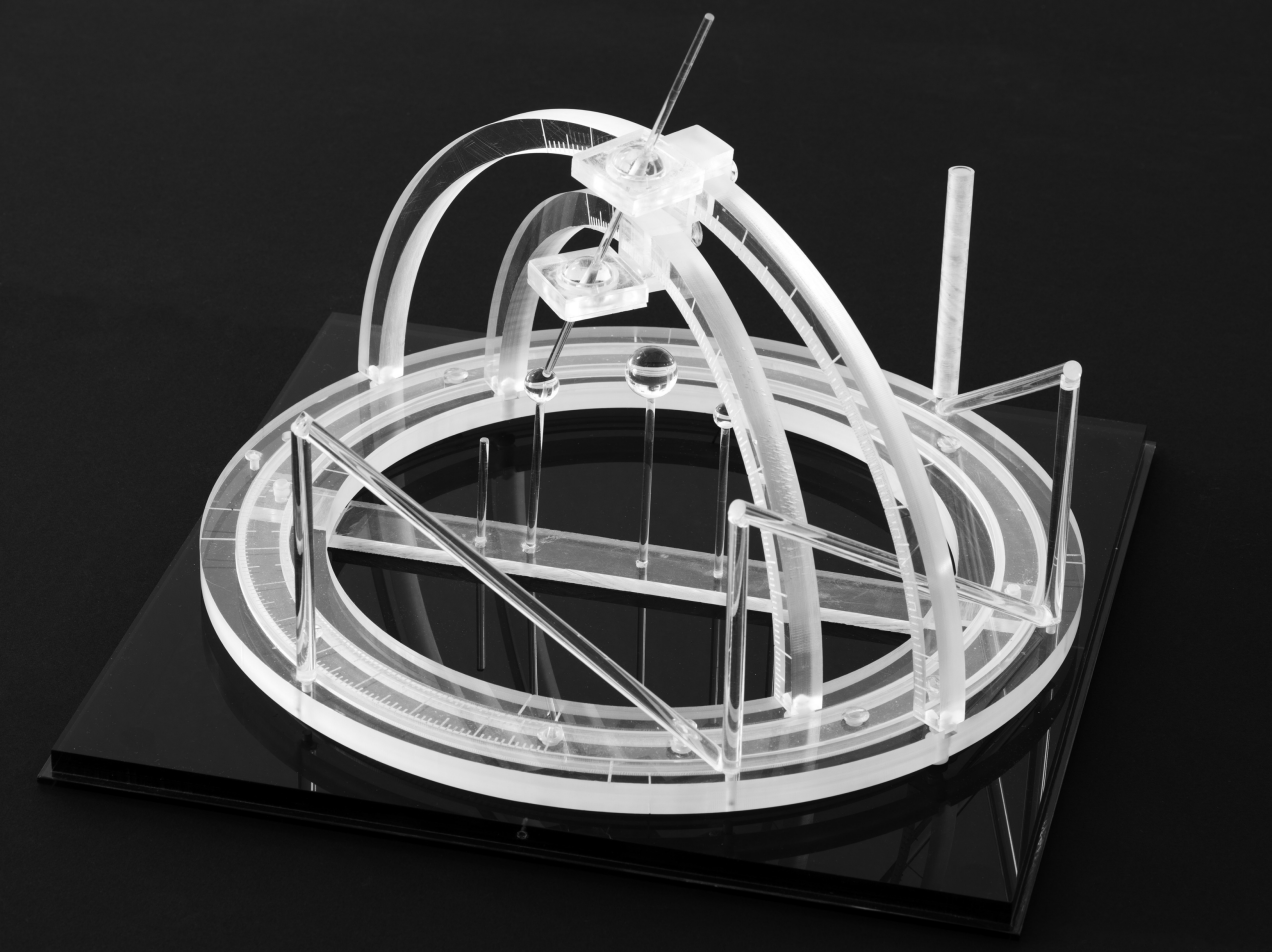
The First CT-Compatible Stereotactic Frame Brown designed and built this stereotactic frame in August 1978 in order to test the concept of the N-localizer [1]. Three N-localizers are attached to this frame and are merged end-to-end such that only seven rods are present. Because three points determine a unique plane in three-dimensional space, the locations of the centers of the three ellipses that the three N-localizers produce in a tomographic image precisely determine the position of the tomographic section with respect to the stereotactic frame [2].
